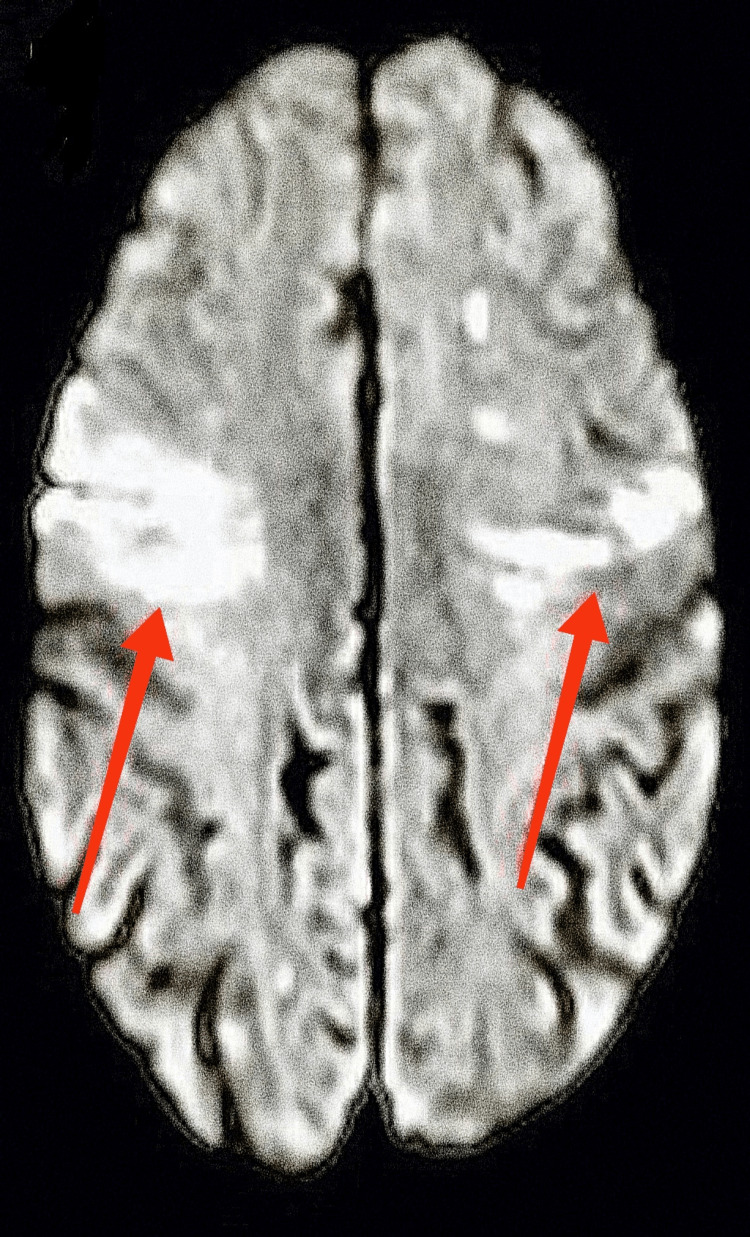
MRI of the head without contrast demonstrating abnormal restriction in the bilateral frontotemporal regions. MRI, magnetic resonance imaging
